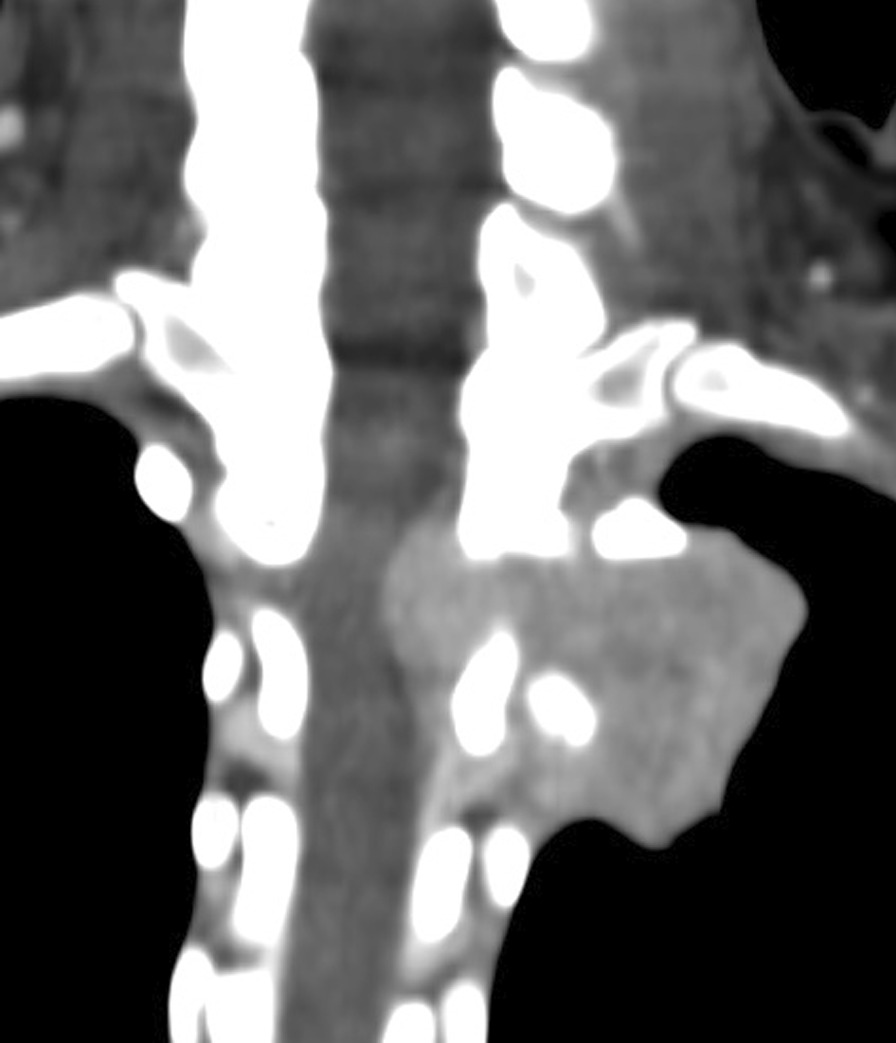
Contrast-enhanced coronal computed tomography (CT) imaging showing a large avidly enhancing left posterior mediastinal paraspinal soft tissue mass with medial extension into the second thoracic vertebral body and further extension into the intervertebral neural foramina, resulting in spinal cord abutment
